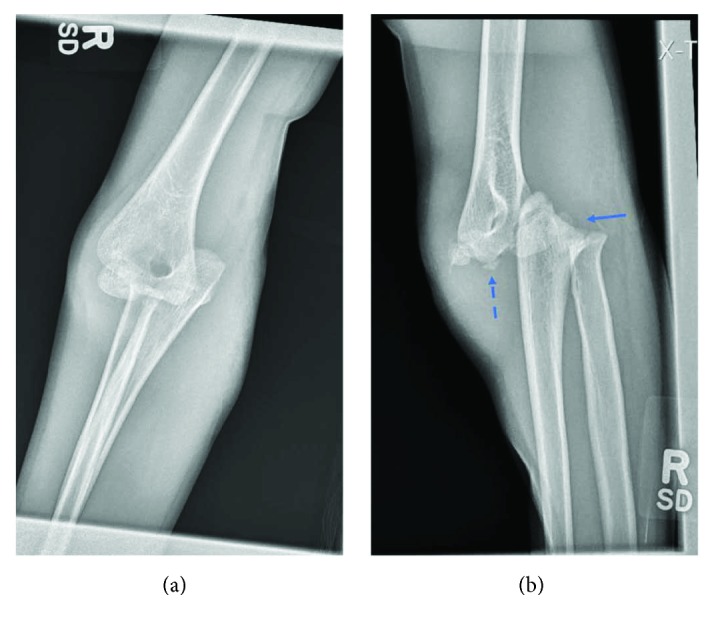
AP (a) and attempted lateral (b) radiographs of the right elbow demonstrating anteromedial elbow dislocation in a skeletally immature individual. The solid arrow points to the Salter-Harris radial head fracture with significant radial head displacement. The dashed arrow points to an ossific fragment near the distal humerus which likely represents the olecranon avulsion.
